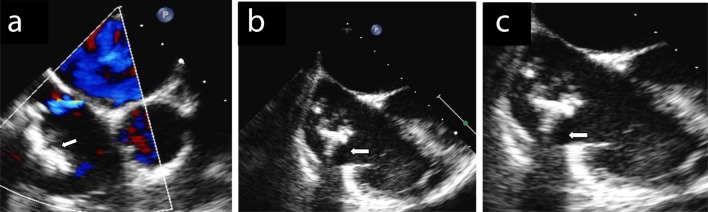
(a, b, c) Transesophageal echo confirmed the location and the extent of the lesion and showed a large patent foramen ovalis through which a left to right shunt was detected after infusion of contrast media in basal conditions and right to left shunt after Valsalva maneuver.
